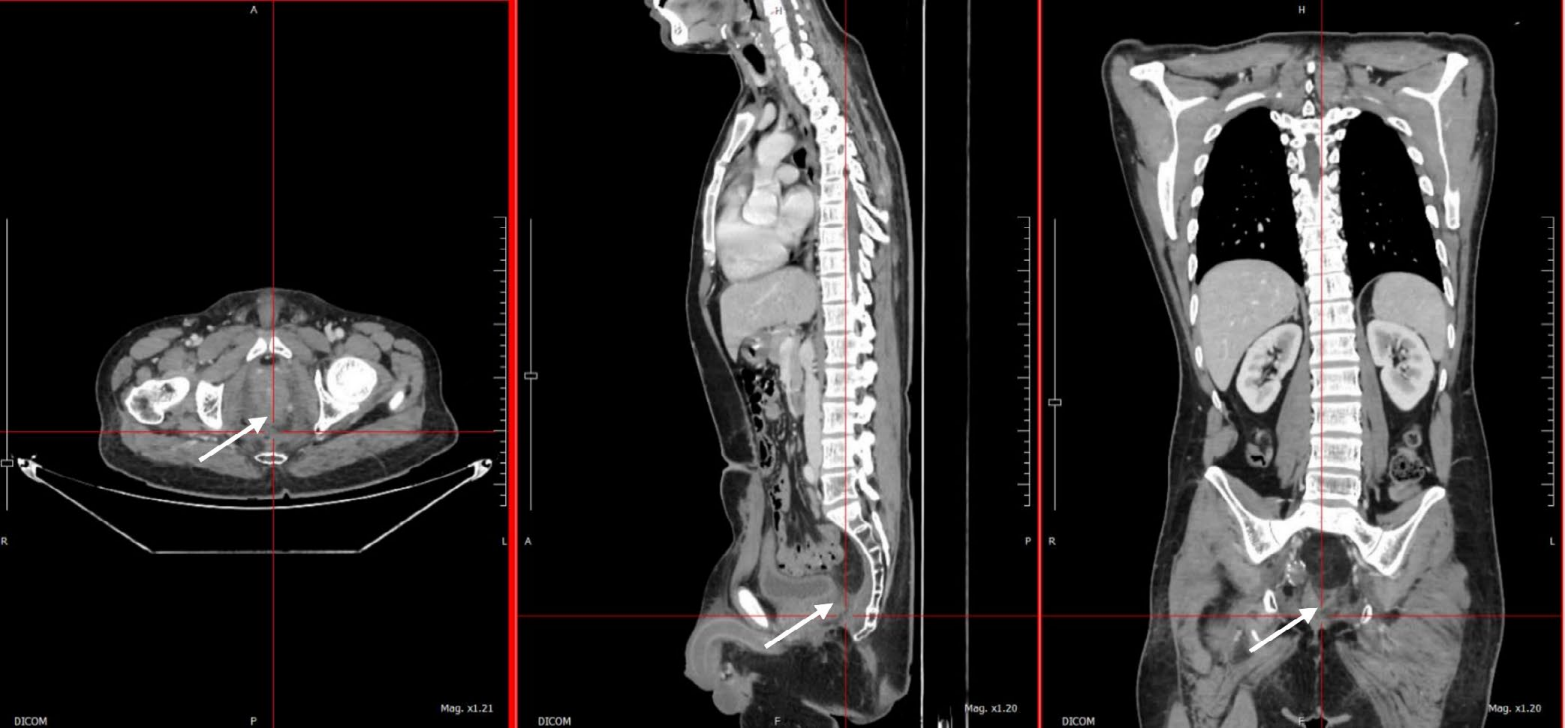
Contrast‐enhanced computed tomography of abdomen and pelvis showing no abnormal enhancement at the operation site with mildly enhancing thickening in the presacral region—likely postoperative changes without any evidence of disease recurrence and metastasis in a known case of rectal carcinoma (post‐radiochemotherapy and postoperative status).
